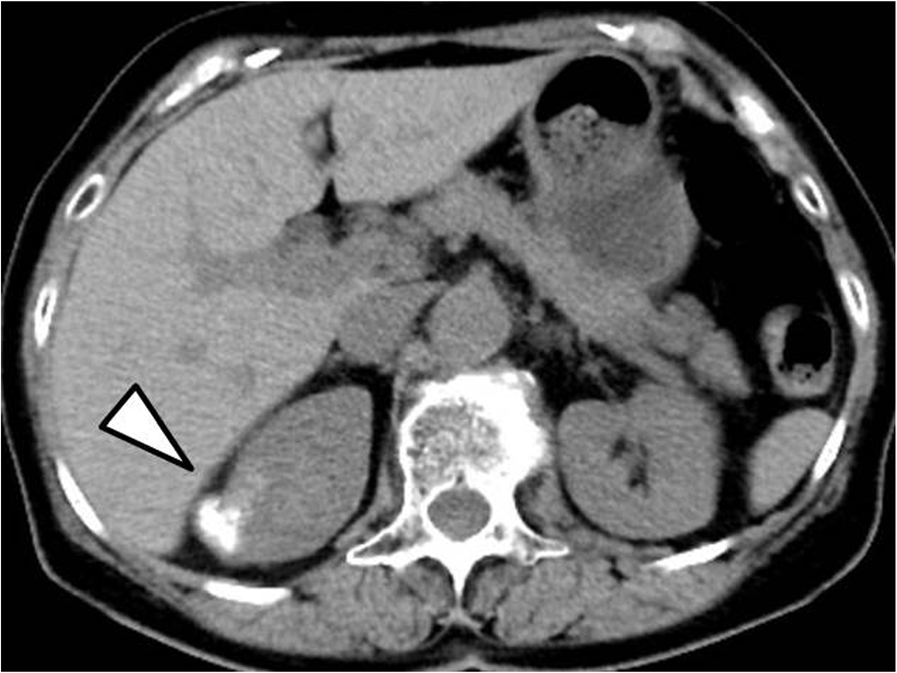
Plain abdominal computed tomography (CT). Abdominal CT revealed a hypovascular tumor with massive calcification, measuring 2.5 × 2.3 cm, in the upper pole of the right kidney (arrow).
